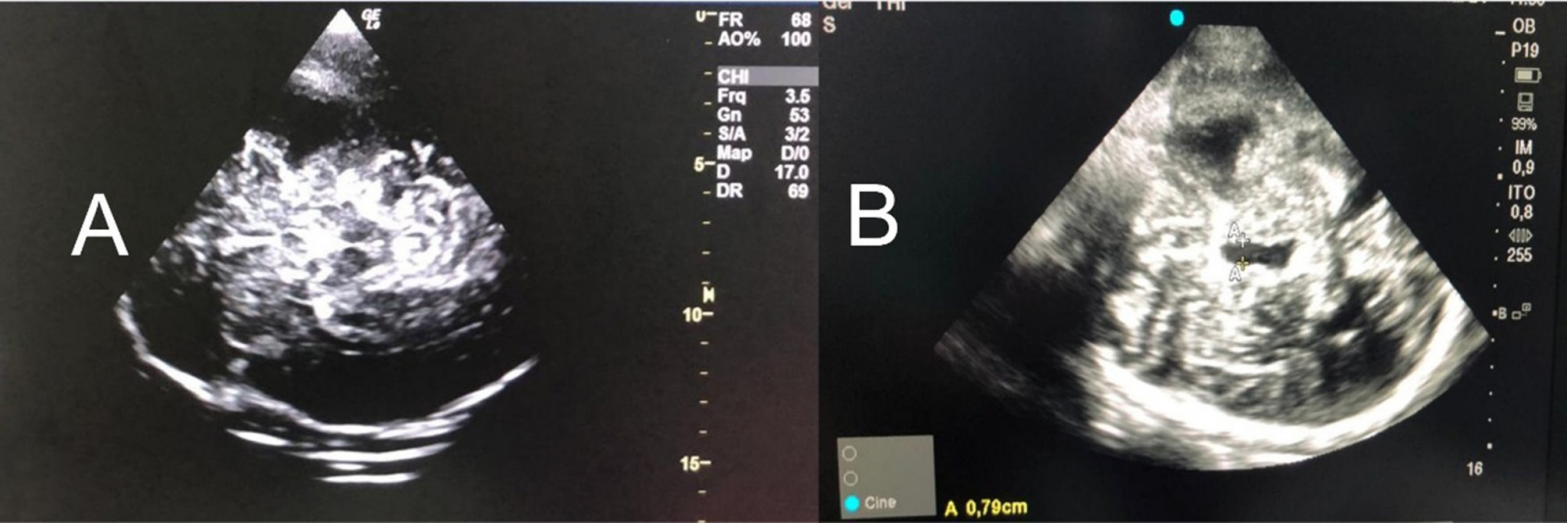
(A) Mesencephalic plane and the imaging of the butterfly-shaped brainstem is a prerequisite to obtain a landmark for orientation [observed in 90–95% of the patients (3–5)]. (B) Tilting the probe 10° cranially, in a diencephalic plane and the third ventricle is obtained (A-A). It should be measured the largest transverse diameter of the third ventricle with its hyperechogenic margins. In addition, the frontal horn of the contralateral lateral ventricle can be measured (5). To ensure the accurate and reproducible measurement of the ventricles' widths, measurements with the US should be performed from the ipsilateral to the contralateral inner layer of the hyperechogenic ependyma. US, ultrasound.
